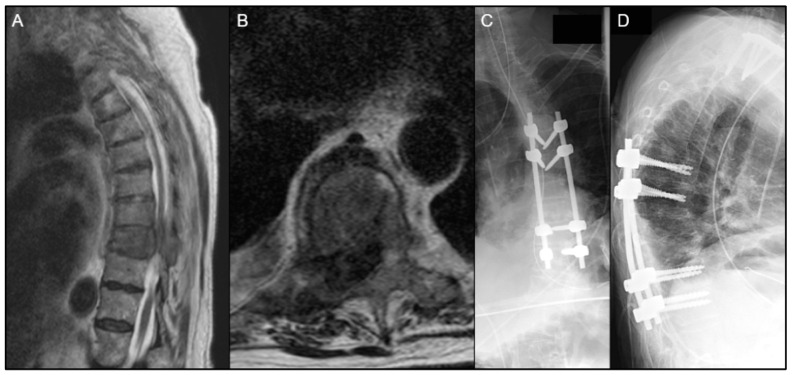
(A–D) A 78-year-old female with a history of stage IV metastatic breast cancer presented with urinary retention and lower extremity numbness within a 4-day duration, who was found to have metastatic tumor causing spinal cord compression with pathologic fracture at T8–T9 on T2-weighted MRI (A,B). She underwent separation surgery with T6–T11 posterior spinal instrumentation and fusion; T8, T9, and T10 laminectomy; right-sided T9 transpedicular decompression; and inferior facetectomies from T6 to T11, as seen on postoperative posteroanterior (C) and lateral (D) X-rays. Intraoperative ultrasound and neuromonitoring were used. The patient received postoperative EBRT after 1 month, and had wound infection 10 days after radiation, which required surgical debridement.
